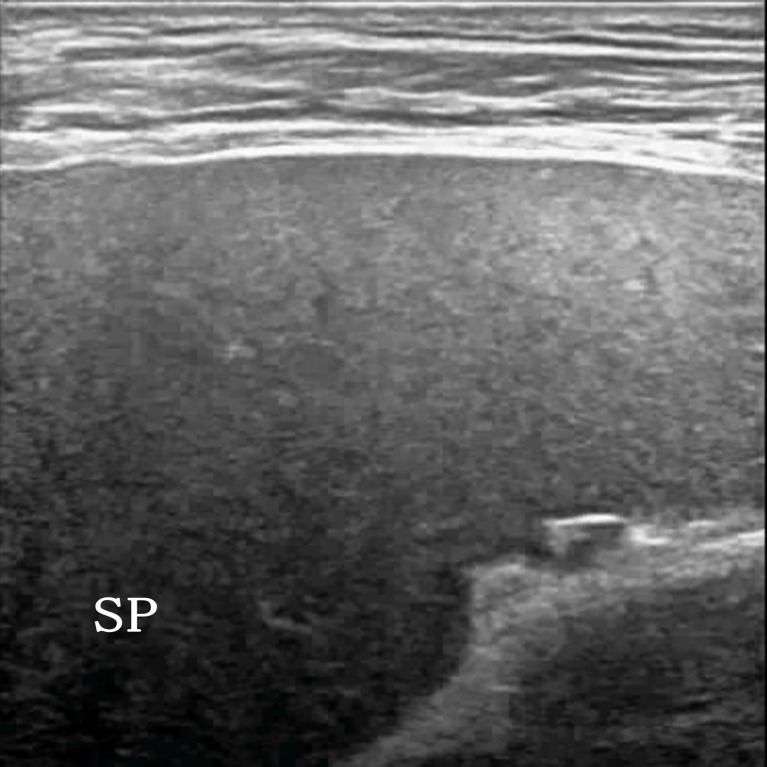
A 54-year-old man clinically suspected of having splenic lymphoma. Two-dimensional ultrasound (2D US) showed uniform splenic echogenicity.
